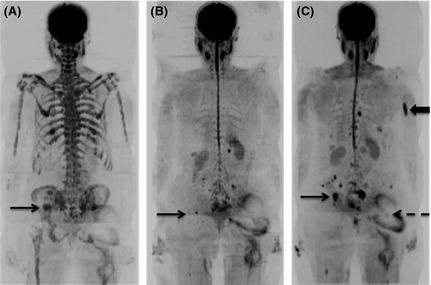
The extremely high sensitivity of diffusion weighted magnetic resonance imaging (DW‐MRI) allows detection of tiny foci of residual disease post‐autograft. b900 whole body DW‐MRI Maximum Intensity Projection images of a 70‐year‐old female with multiple myeloma before (A), 3 months post‐ (B) and 8 months post‐ (C) autograft. At baseline there is widespread marrow disease including a focal lesion in the right iliac bone (A, arrow). At 3 months post‐autograft, the marrow signal has normalized apart from a tiny residual focus of abnormal signal at a site of original disease in the right iliac bone (B, arrow). WB DW‐MRI 8 months post‐autograft shows progression of the residual disease (C, arrow) and development of new sites (example shown by block arrow). Ill‐defined distortion overlying the left hip (dashed arrow) represents artefact from a metal hip prosthesis.
